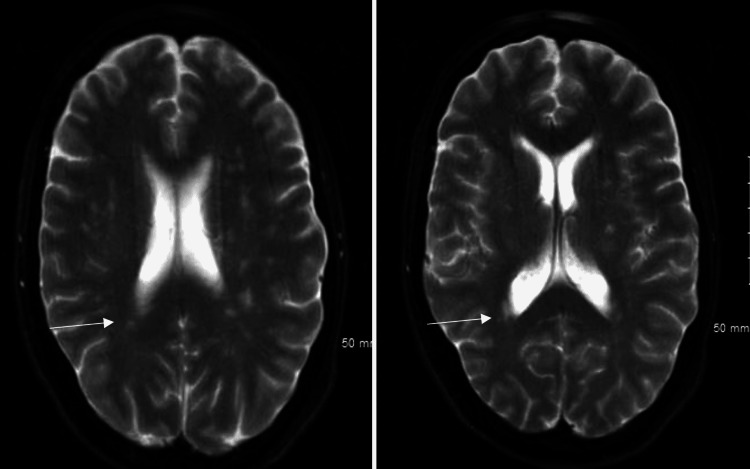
MRI of the head in transverse plane showing periventricular hyper-intensities.
